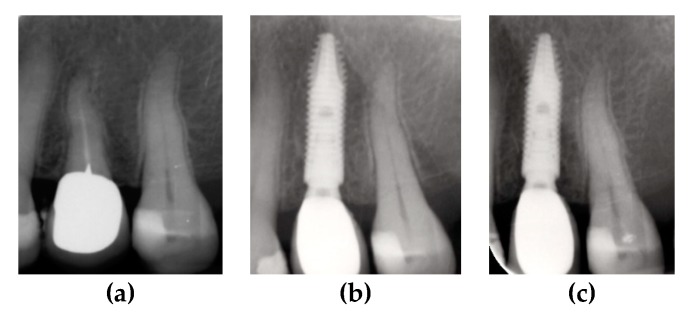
Periapical X-rays showing marginal bone level of CSR dental implant with a reduced neck. (a) Pre-operative X-ray; (b) post-operative follow-up at 12 months; and (c) post-operative follow-up at 24 months.
